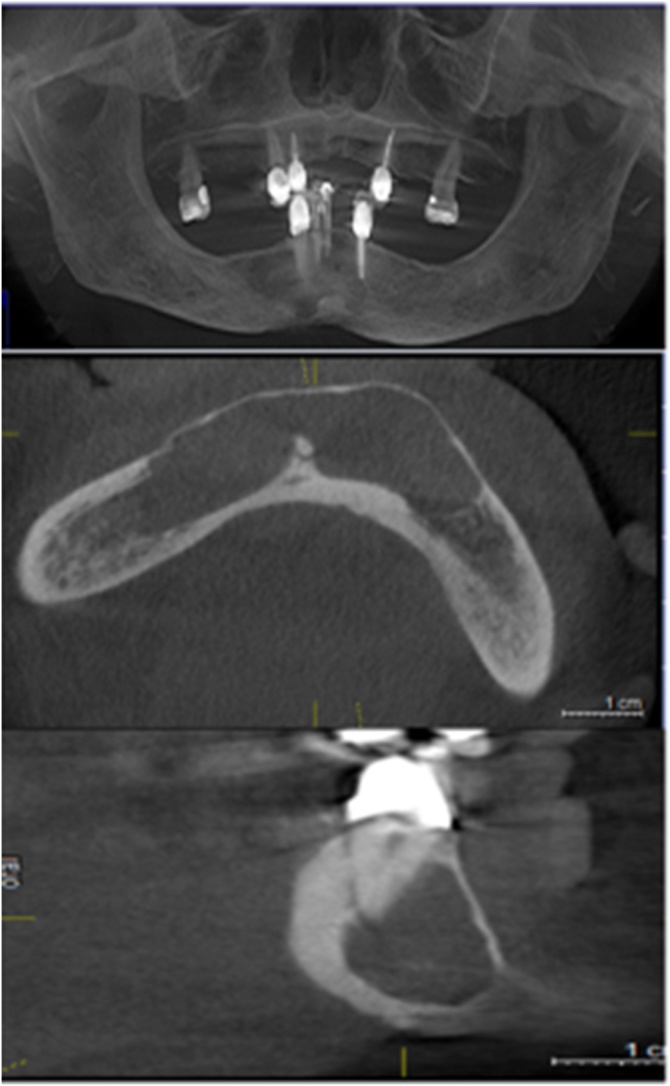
Orthopantomography and CT image showing frontal and sagittal slices of the tumor region.
